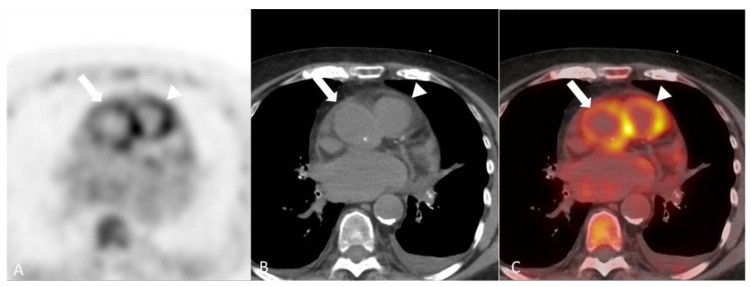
A 75-year-old woman with Behcet’s disease. Axial images of attenuation corrected F-18 FDG PET (A), CT without contrast (B) and fused PET/CT (C) demonstrating concentric tracer avid mural thickening to the ascending aorta involving the root (arrow) as well as the main pulmonary artery (arrowhead), consistent with active vasculitis.
